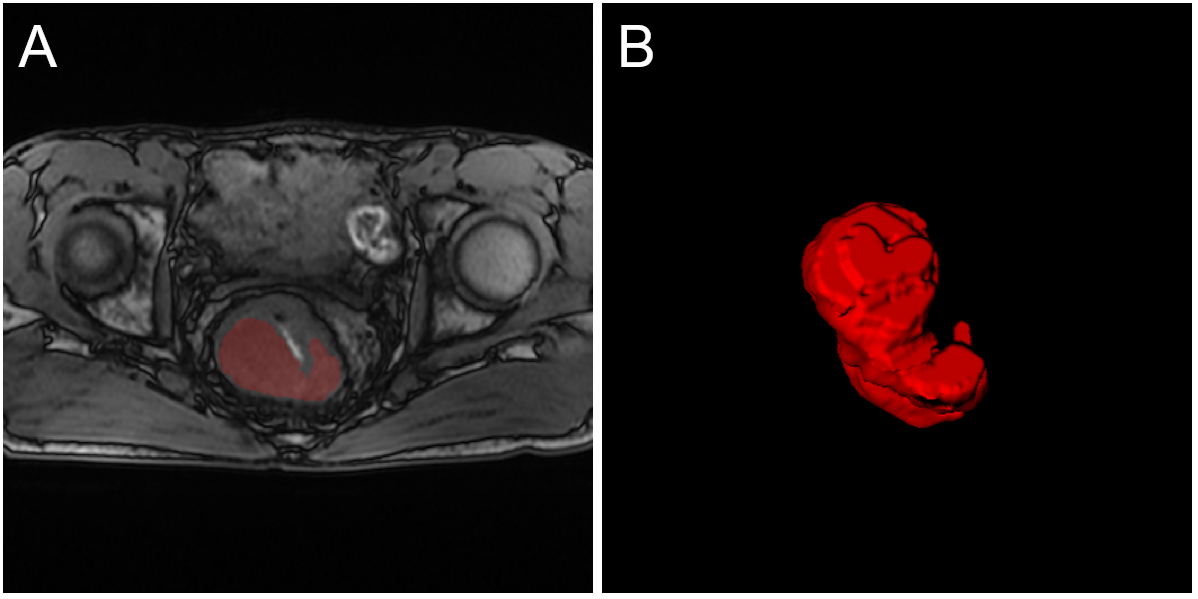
An example of tumor segmentation from T1 MRI. (A) The original image and tumor ROI; (B) The three-dimensional display of the entire tumor area.
